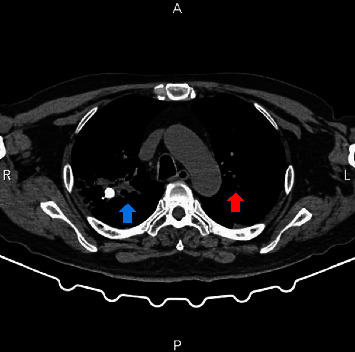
Thorax-computed tomography shows infiltrate in the upper right lobe (blue arrow) and the upper left lobe (red arrow).
